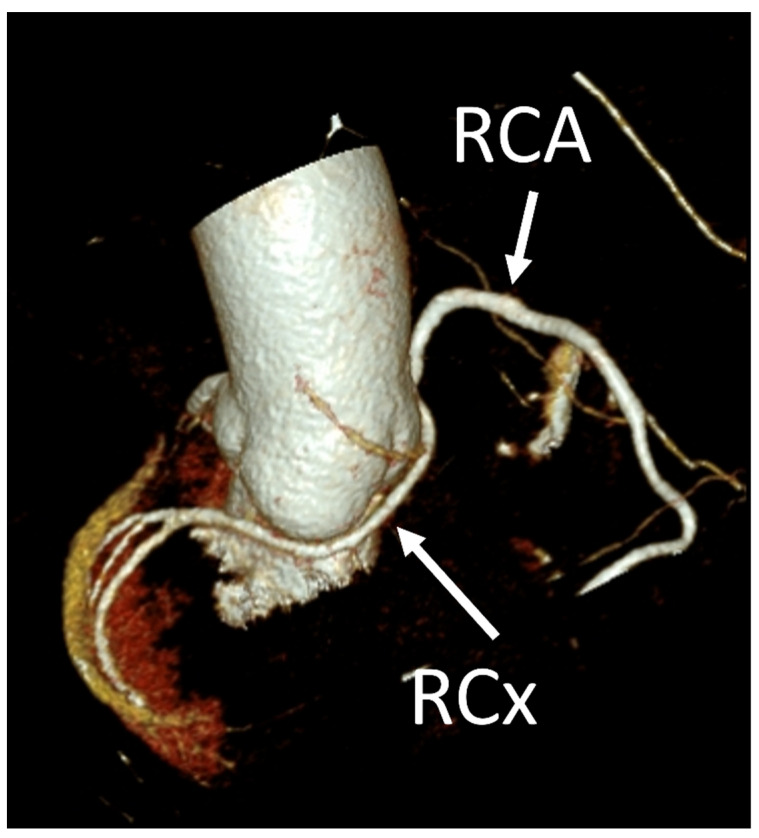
VRT (Volume Rendering Technique) CT image showing the anomalous origin of the left circumflex artery from the right sinus of Valsalva (RCx) in the same patient.
